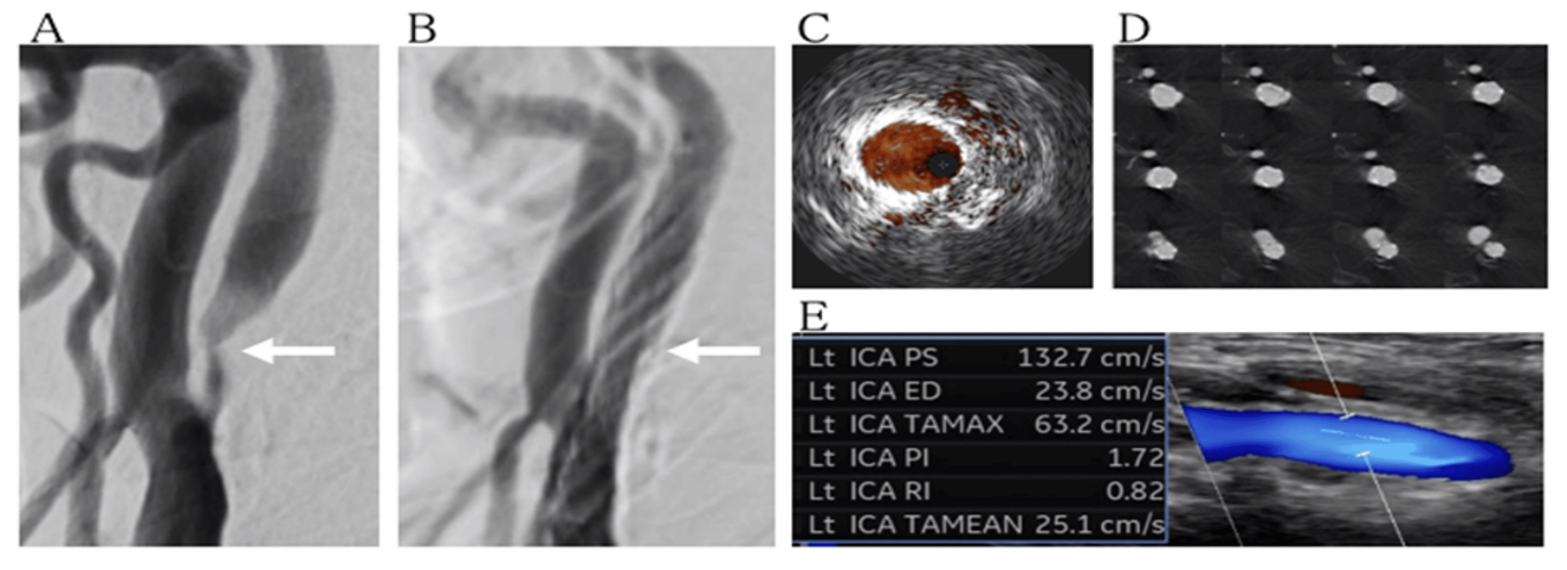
Pre- and post-stenting imaging of the left internal carotid artery. Lateral angiography reveals severe stenosis of the left internal carotid artery (arrow; A). Post-stenting angiography confirms good coverage and no residual stenosis (arrow; B). Intravascular ultrasound reveals a minimum lumen diameter >3 mm (C). 3D angiography shows no plaque protrusion (D). Postoperative carotid ultrasound shows no intrastent plaque and improved flow velocity (E). Lt: left, ICA: internal carotid artery, PS: peak systole, ED: end diastole, TAMAX: time-averaged maximum velocity, PI: pulsatility index, RI: resistance index, TAMEAN: time-averaged mean velocity.
